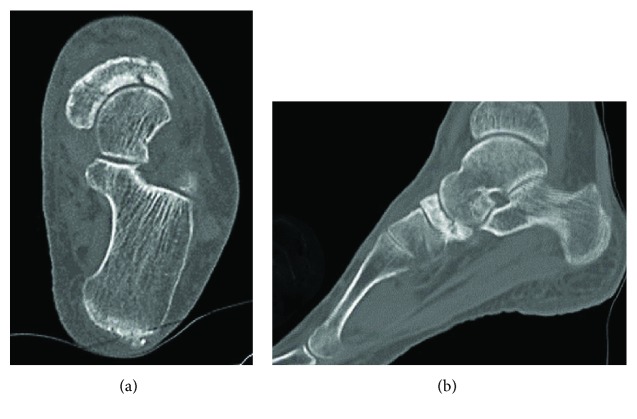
Axial and sagittal CT scans show diffuse sclerosis of the tarsal navicular.
